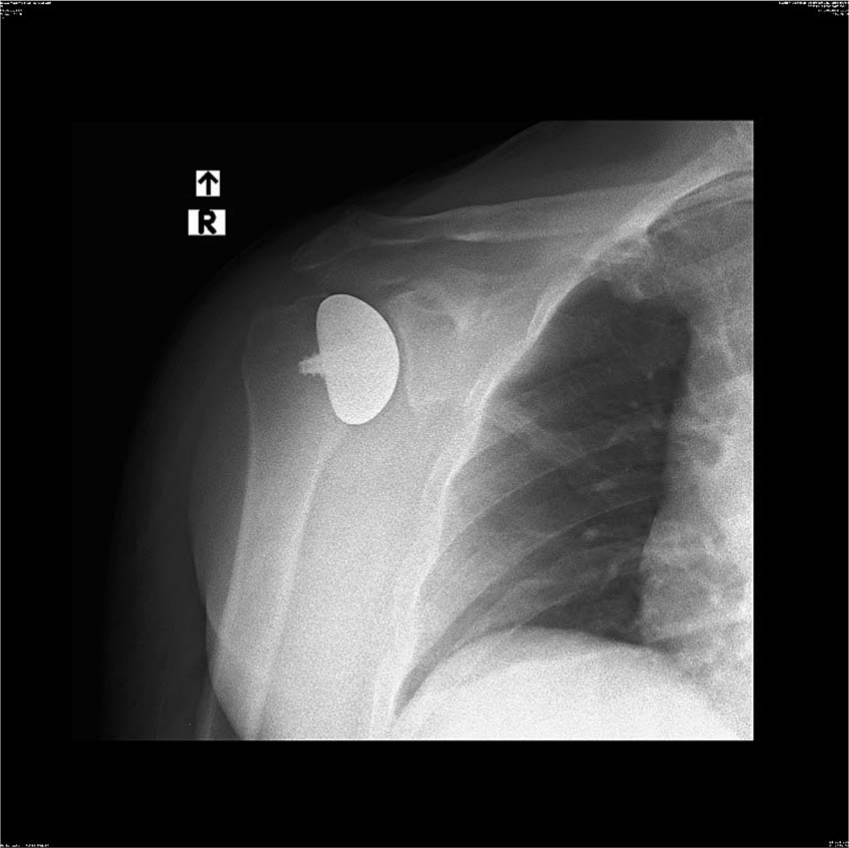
X-ray image after the surgery.
